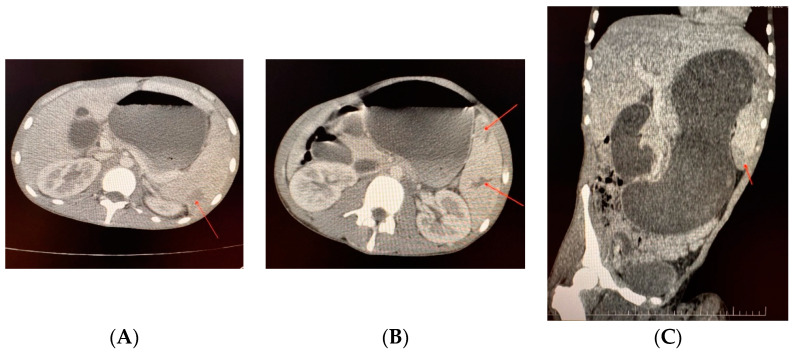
(A–C) Case 3—Abdomen and pelvis computed tomography scan with contrast (anteroposteriorly and sagittal view). Hepatosplenomegaly. Right liver lobe of 20 cm, left liver lobe at the axillary line. The kidneys and pancreas were normal. Gastric dilatation. Spleen enlarged 15/6/19 cm, with mid-body capsular fissure, two hypo-capturing areas at the inferior pole (9/2/3 cm) and posterior median region (3/3 cm), and subcapsular hematoma. Without abdominal-pelvic collections.
